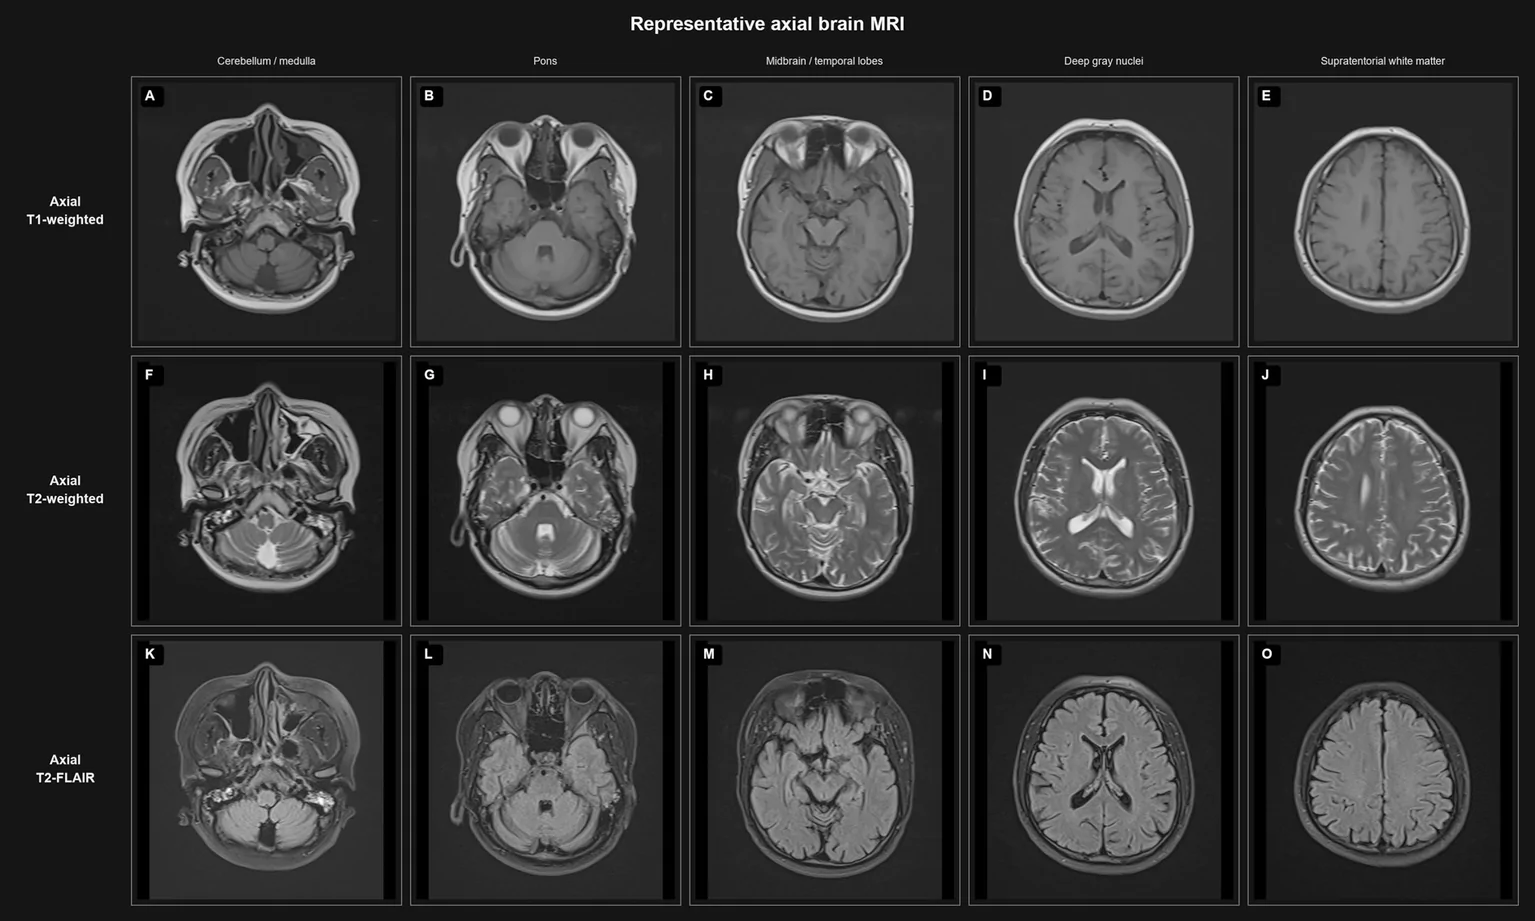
Representative axial brain magnetic resonance imaging findings in Patient 1. (A–E) Axial T1-weighted images showing the cerebellum, pons, midbrain, deep gray nuclei, and supratentorial regions, respectively. (F–J) Axial T2-weighted images corresponding to the same anatomical levels. (K–O) Axial fluid-attenuated inversion recovery (FLAIR) images corresponding to the same regions. Mild enlargement of the bilateral lateral ventricles and slight widening and deepening of several cortical sulci were observed. MRI, magnetic resonance imaging; FLAIR, fluid-attenuated inversion recovery.
